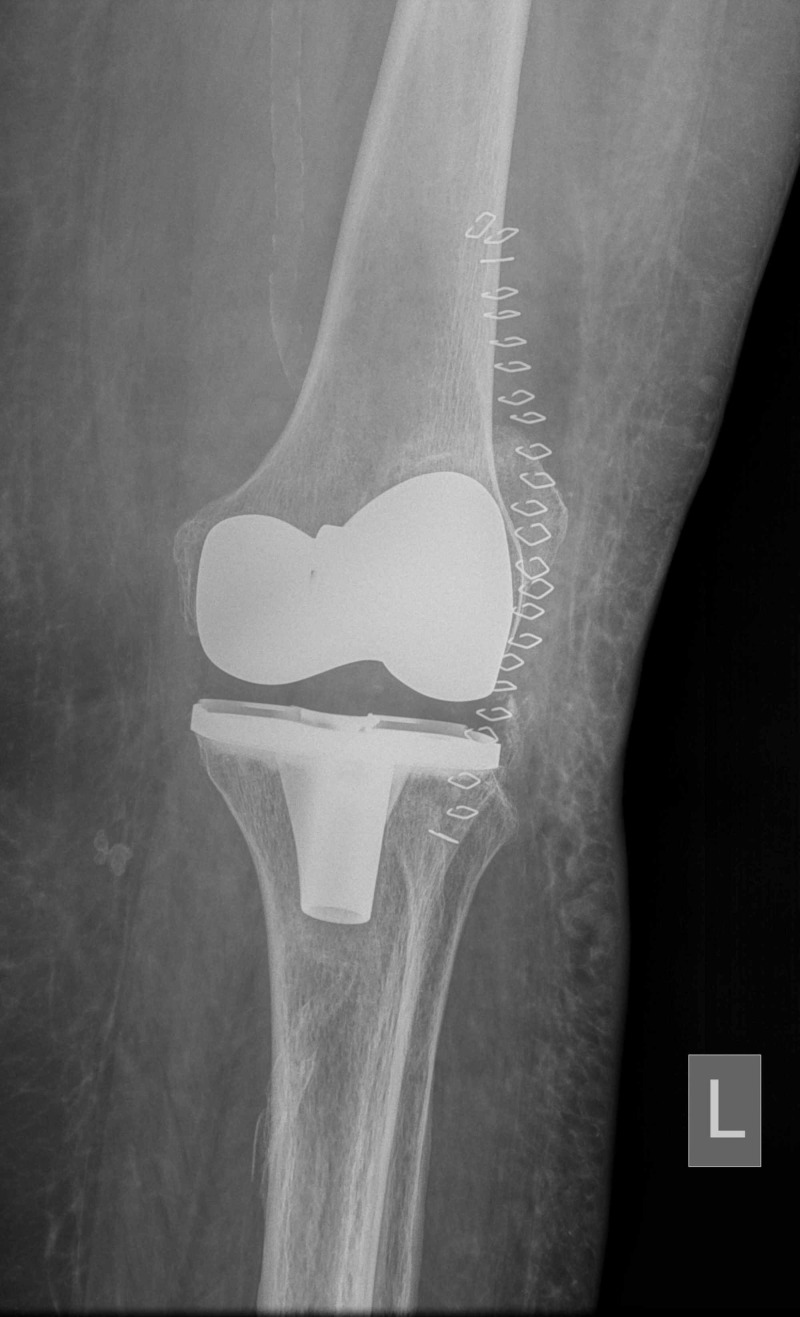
Immediate postoperative anteroposterior left knee radiograph L: left
